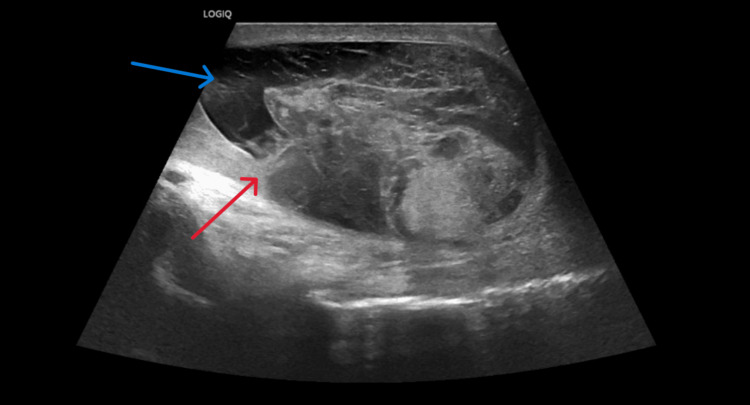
Sagittal grayscale ultrasound of the right testicle approximately 18 hours after injury. Exam shows contour irregularity and tunica albuginea disruption (red arrow), hematoma collection (blue arrow), and heterogenous echotexture. These findings are consistent with a radiological diagnosis of right testicular rupture.
